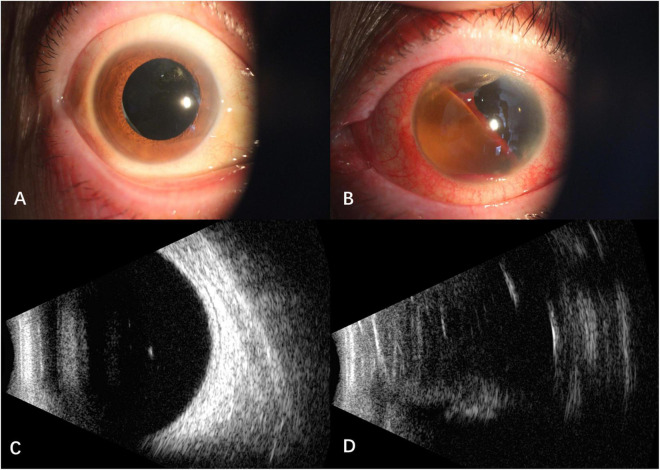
Anterior segment and B -scan ultrasonography of the patient 5 weeks after the onset of intraocular hemorrhage. (A) Hemorrhage in the anterior chamber of the right eye absorbed; (B) intraocular lens was removed and the hemorrhage absorbed in the left eye; (C) no vitreous hemorrhage in the right eye; (D) vitreous silicone oil filling with retinal detachment in the left eye.
